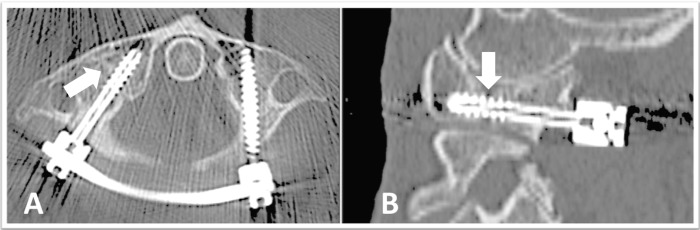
Final postoperative images A follow-up computed tomography (CT) scan at six months after the second stage with (A) axial and (B) sagittal views demonstrating a stable C1 screw-rod construct with evidence of bone growth across the fracture
